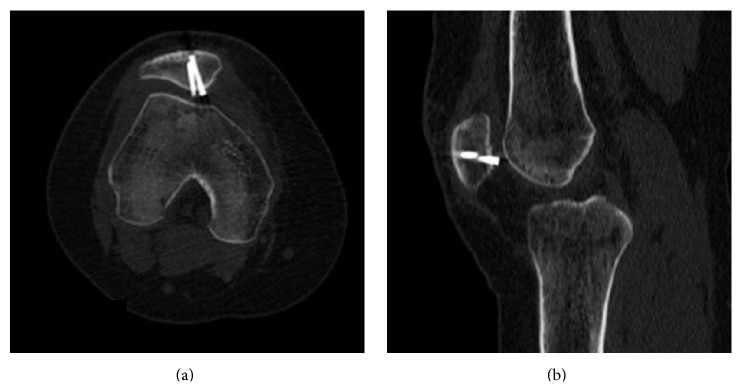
CT showing the relationship between the patellofemoral joint and implant: axial (a) and sagittal (b) slices.
